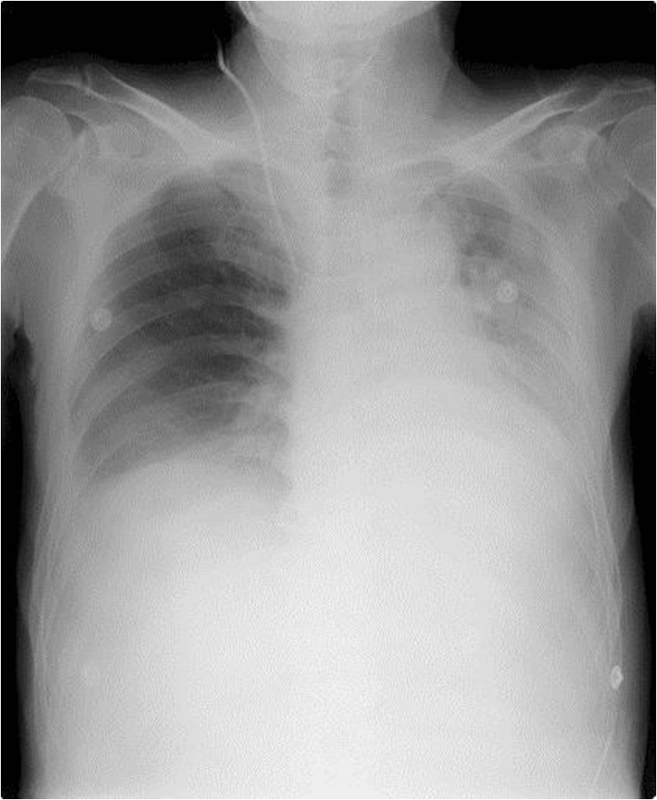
Chest X-ray on POD 7 shows massive atelectasis in the left lower lobe.
